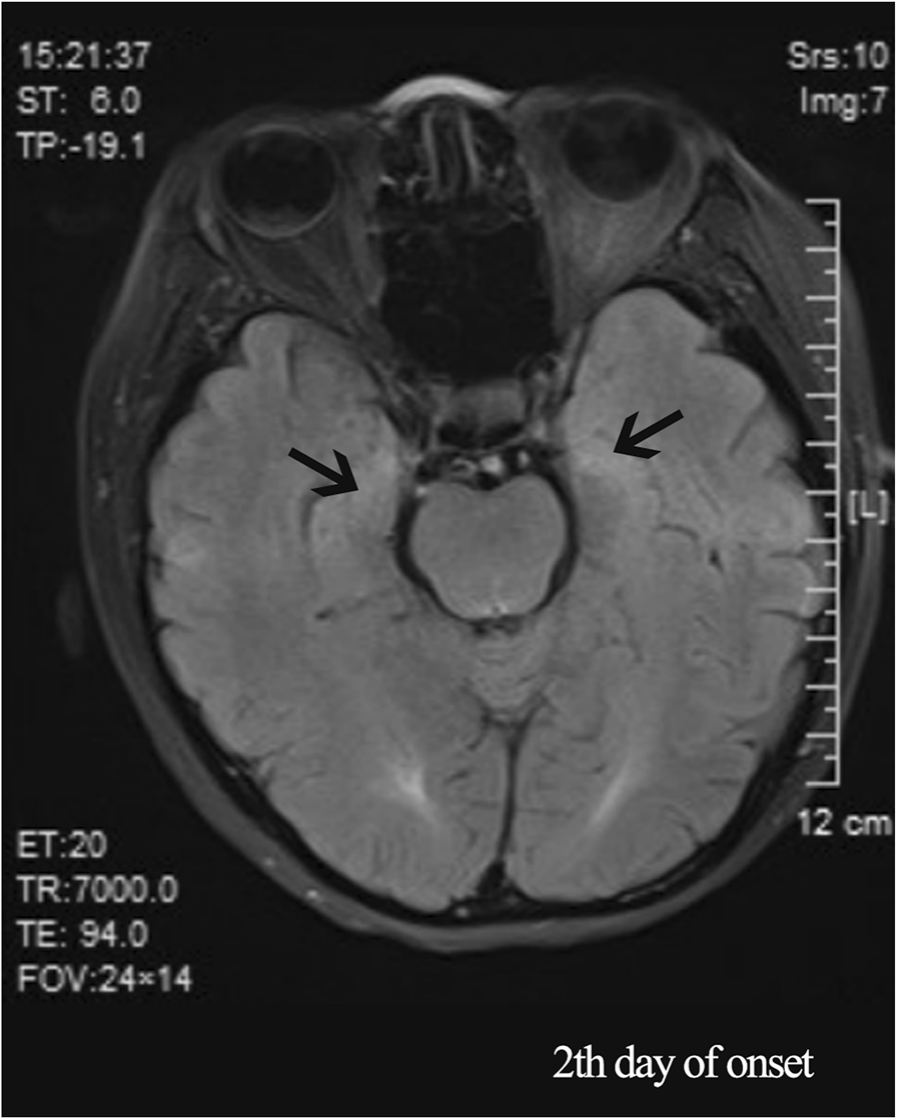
Brain MRI showed fluid attenuated inversion recovery (FLAIR) hyperintense lesions (white arrows) in bilateral cingulate gyrus and bilateral temporal cortex
